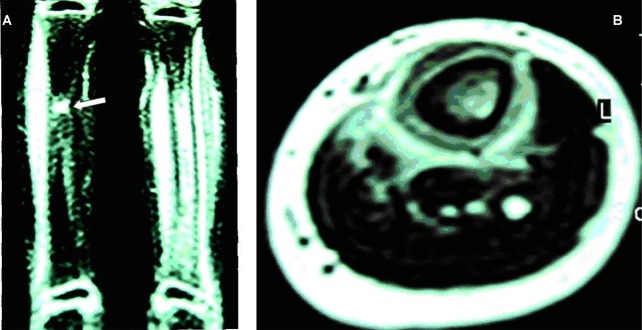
A. Coronal STIR MRI demonstrated tibial marrow edema in the shaft and surrounding soft tissue, also note minor edema of right tibia were in favor of osteomyelitis. The arrow shows site of previous bone biopsy of right tibia. B. Axial T1 weighted image of left leg reveal decrease in signal intensity in the tibial marrow, periosteal elevation and edema of soft tissue.
